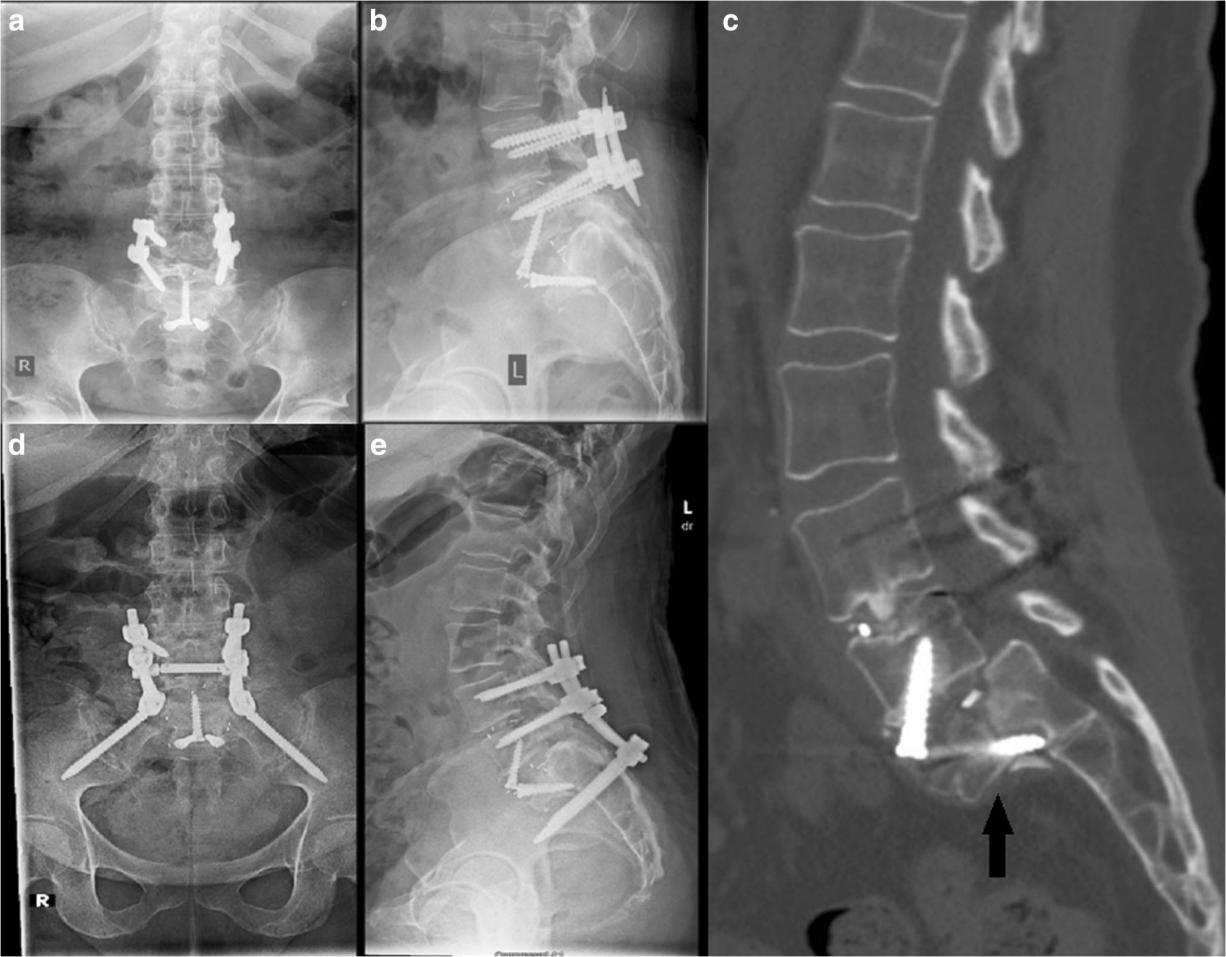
a Anteroposterior (AP) and b lateral plain radiographs taken before sacral fracture. c Representative lateral computed tomographic cut showing sacral fracture (black arrow). d AP and e lateral post-operative plain radiographs.
